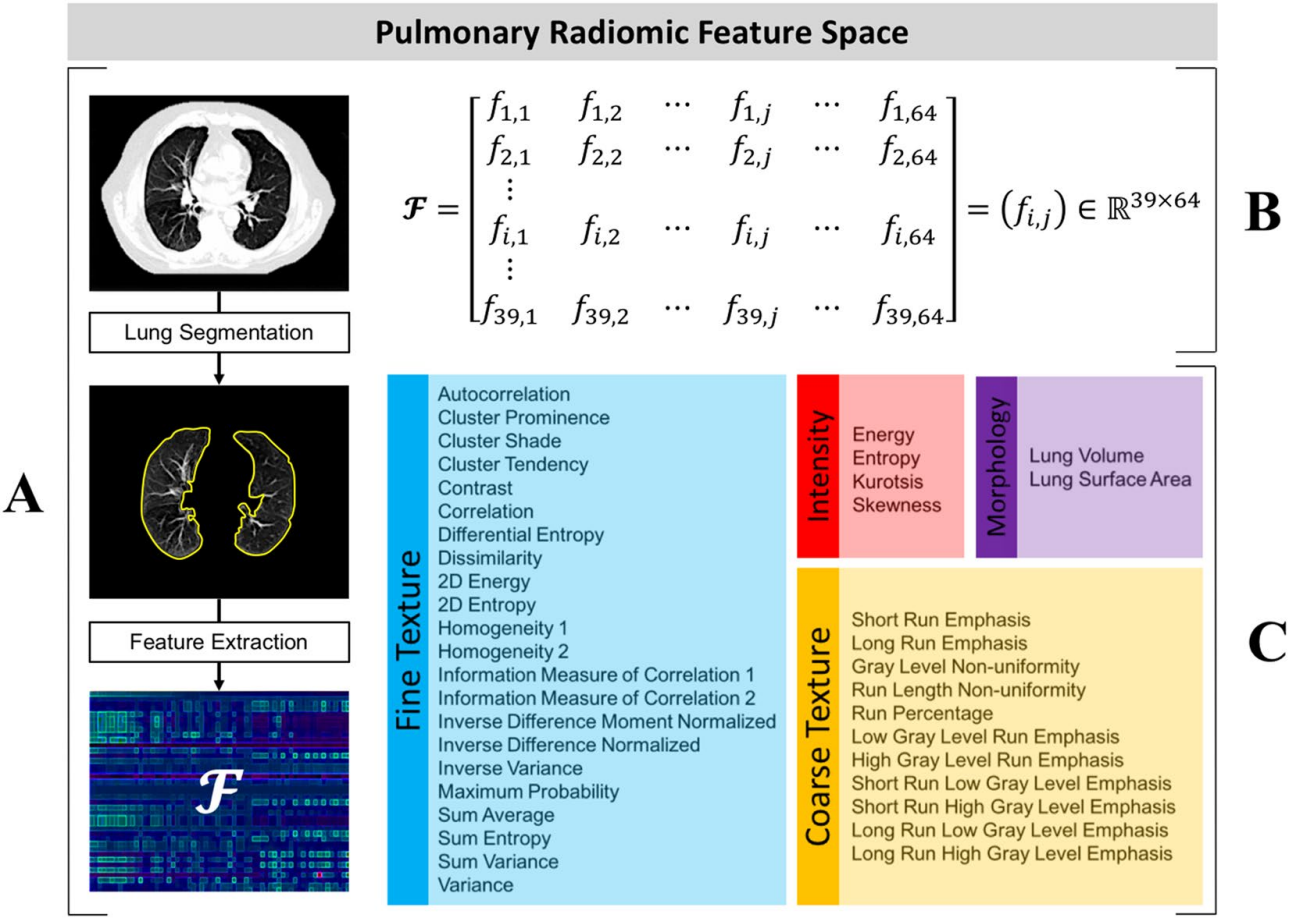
Pulmonary radiomic feature space design. (A) Radiomic features are extracted from CT-segmented lungs to derive a feature space, $${\boldsymbol{ {\mathcal F} }}$$. (B) $${\boldsymbol{ {\mathcal F} }}$$ is mathematically defined as a (39 x 64)-dimensional matrix. The $${(i,j)}^{th}$$ coordinate of $${\boldsymbol{ {\mathcal F} }}$$ represents the measured value of the *i*^*th*^ feature as observed within the image of the *j*^*th*^ patient. (C) The features used to construct $${\boldsymbol{ {\mathcal F} }}$$, color coded by class.
